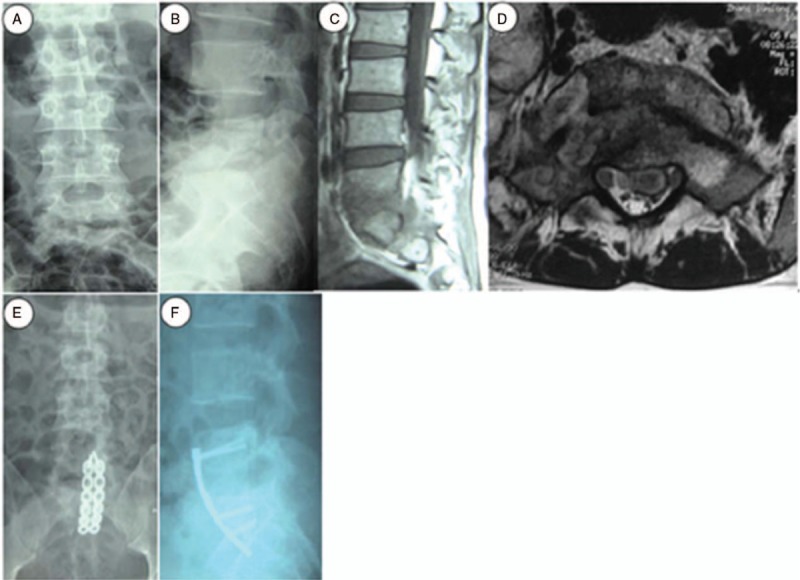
A 28-year-old woman underwent anterior debridement and reconstruction with anatomical screw-plate fixation for L5–S2 TB in our hospital. Preoperative, posteroanterior (A) and lateral (B) plain radiographs showed the L5–S2 vertebral damage with a narrowed intervertebral space and a decreased lumbosacral angle. The sagittal (C) and coronal (D) MRI views showed bony destruction from the L5 to S2 vertebrae, with prevertebral and paravertebral abscesses compressing the neural elements. Anteroposterior (E) and lateral (F) immediate postoperative radiographs showed the anterior column reconstruction with two 5.6-cm-long anatomical screw-plates across L5–S2.
